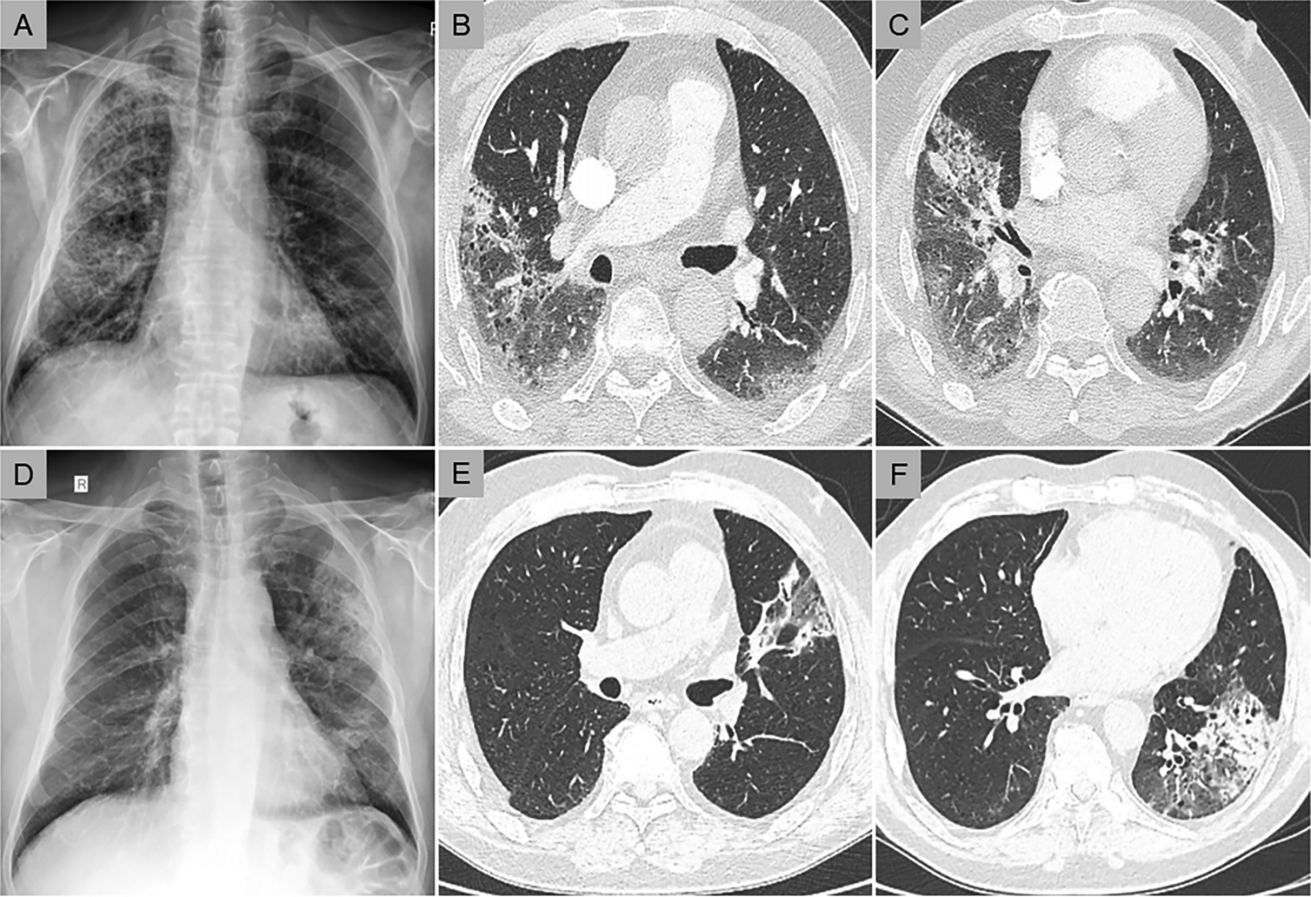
Radiological findings showing a migratory pattern of pulmonary infiltrates. (A) Admission chest radiograph demonstrating bilateral alveolar infiltrates, more prominent on the right, with subtle interstitial prominence. (B, C) High‐resolution computed tomography images at presentation revealing bilateral peripheral consolidations and ground‐glass opacities. (D–F) Earlier imaging showing pulmonary infiltrates that later resolved, whereas new lesions subsequently developed in different lung regions, consistent with a migratory pattern.
